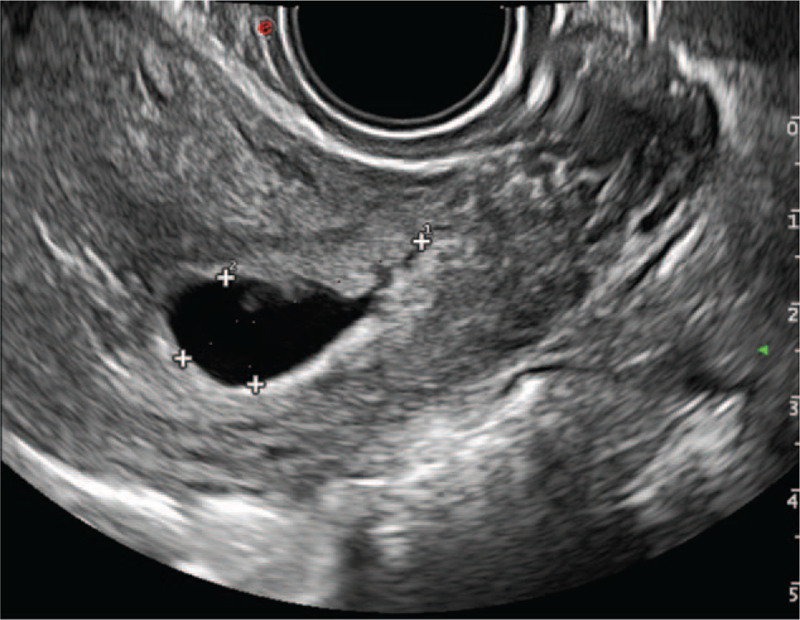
Colour power Doppler image showing the caesarean scar pregnancy in the lower uterine segment.
